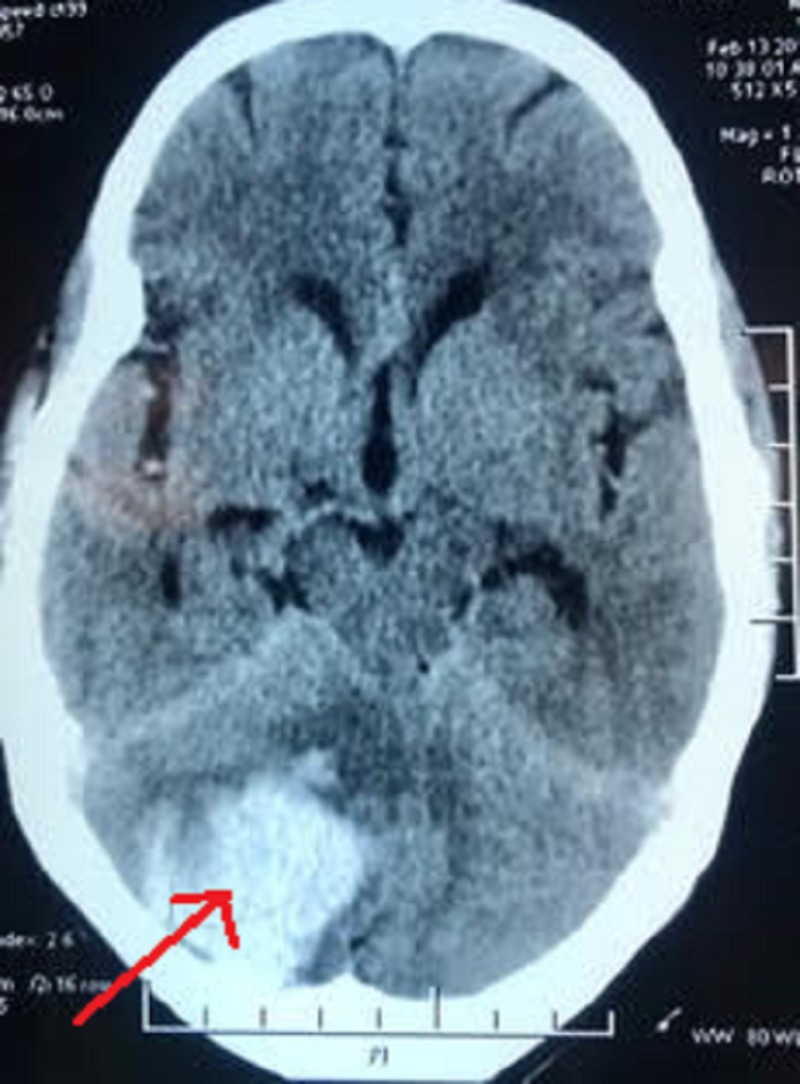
Pre-operative CT head showing right-sided cerebellar hemorrhage with effacement of fourth ventricle
